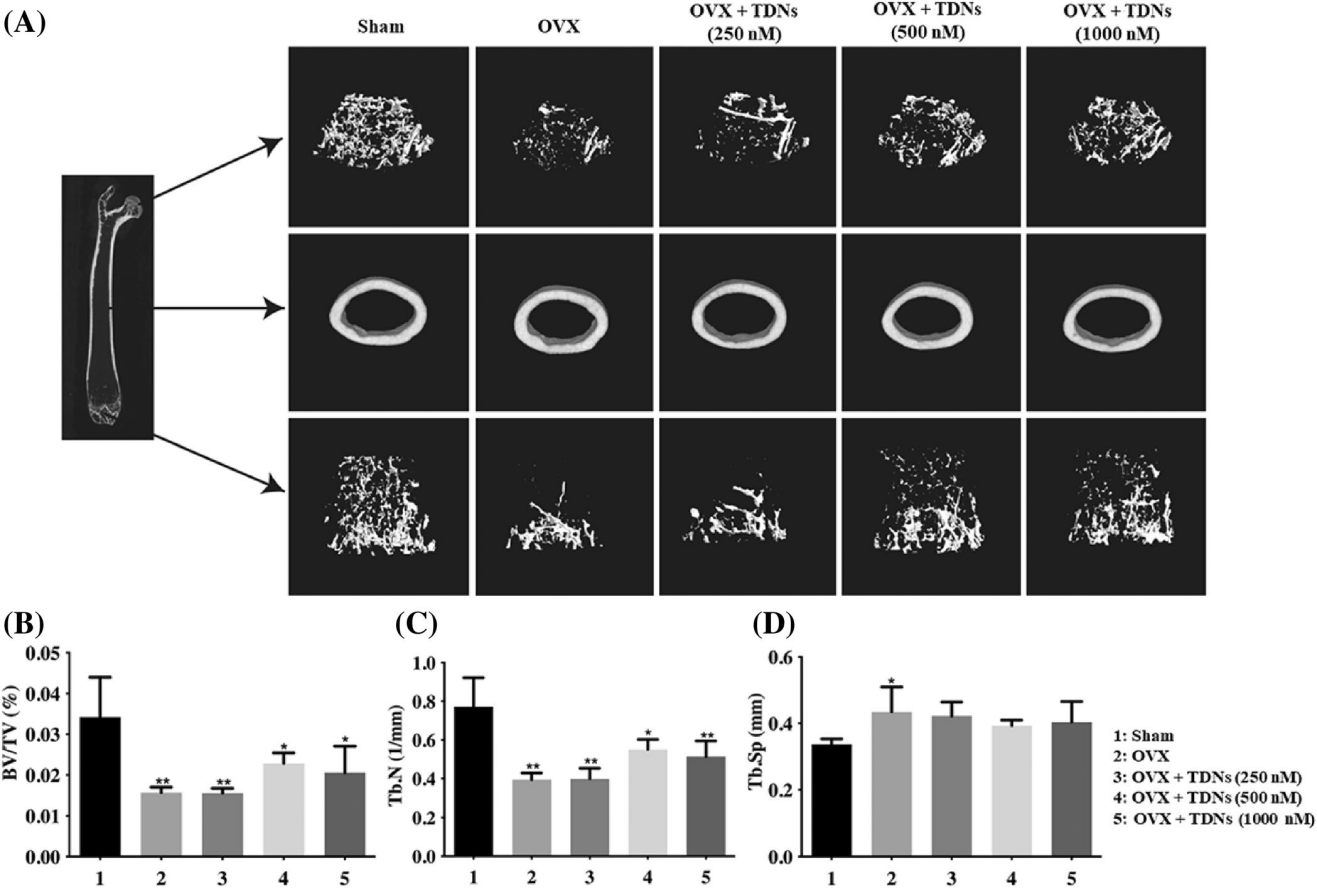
Microcomputed tomography (Micro‐CT) images of the femurs. (A) Micro CT images of the femurs. (B–D) Analysis of bone volume fraction (BV/TV), bone trabecular number (Tb. N), bone separation (Tb. SP) of femurs after treatment with different materials at 2 months, respectively (p* < 0.05, *p* < 0.01 vs. Sham).
